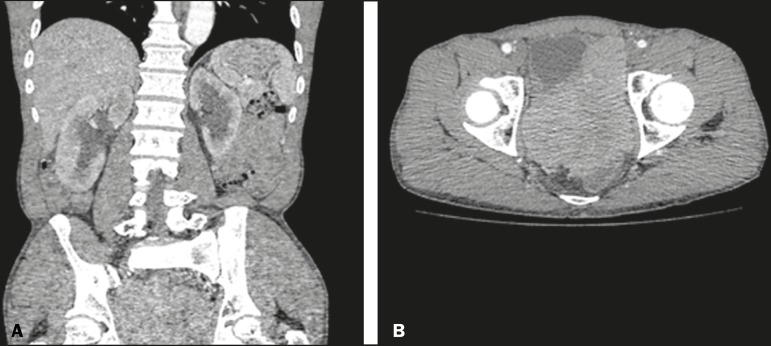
Contrast-enhanced coronal and axial computed tomography of the abdomen
